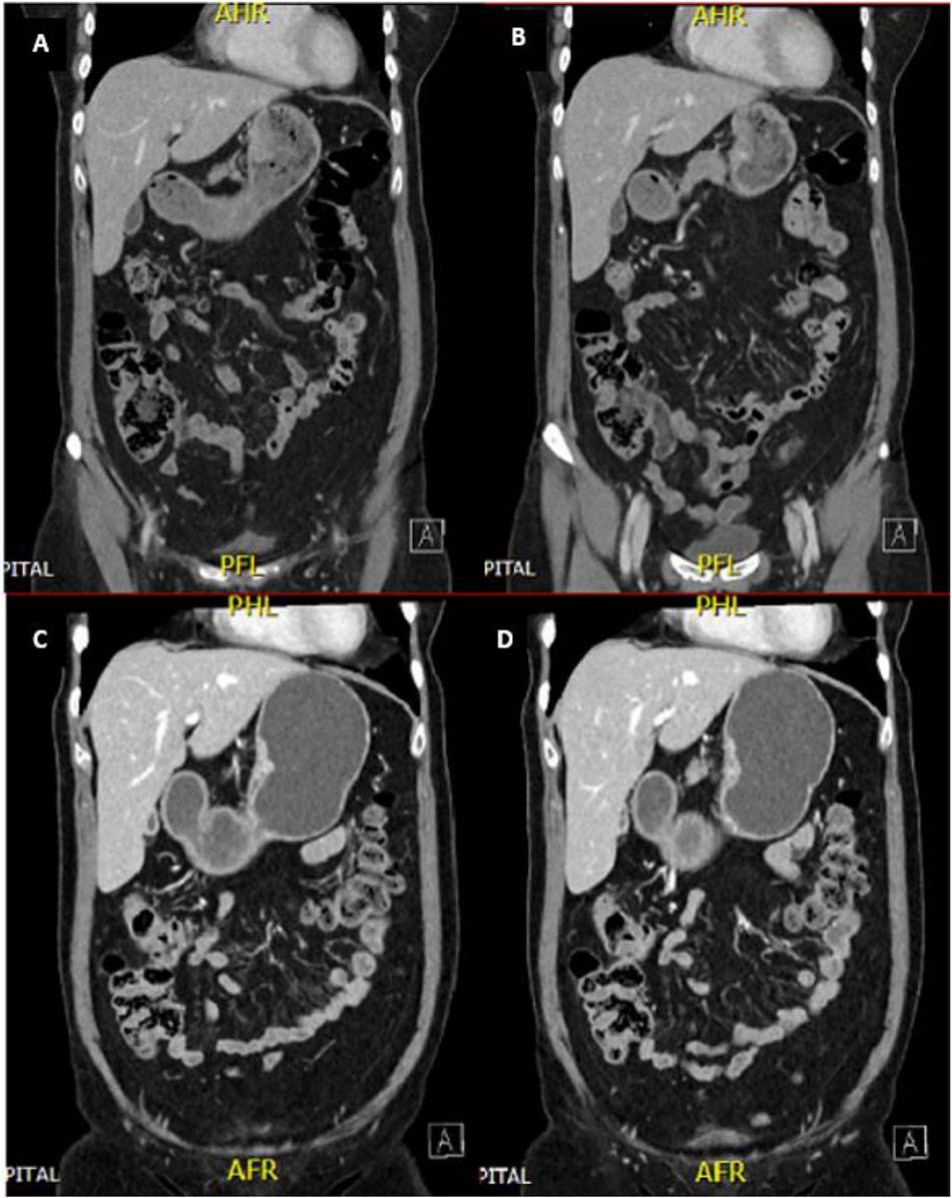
CT abdomen and pelvis demonstrated an enhancing nodular lesion along the gastric lesser curvature (A and B). Interval CT abdomen and pelvis showed reduced gastric wall thickening following 7 weeks of chemotherapy (C and D).
